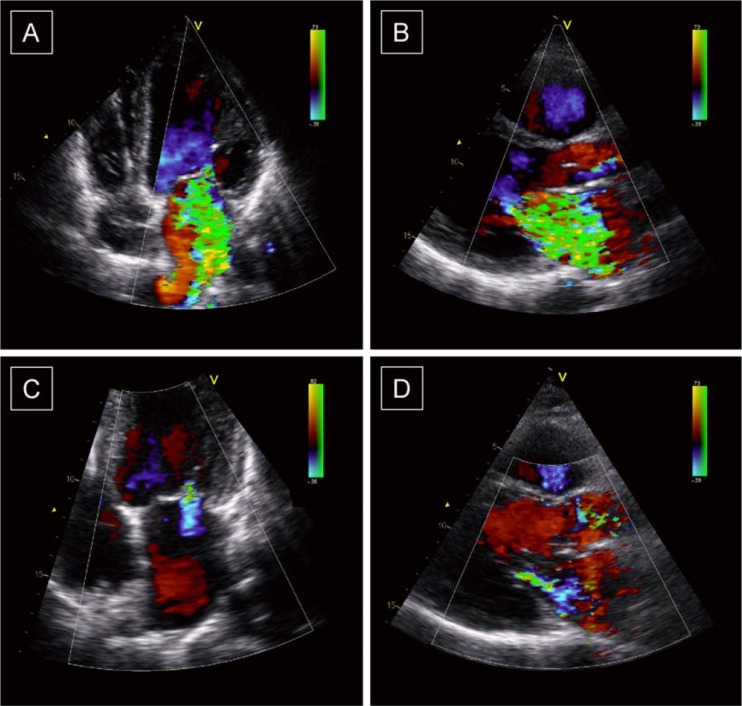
Example of a patient with a significant improvement in mitral regurgitation 6 months after cardiac resynchronization therapy. At baseline, the MR jet area exceeded 80 % of the left atrium area and the effective regurgitant orifice area (EROA) was 0.61 cm^2^ (Panels A [4-chamber view] and B [parasternal long-axis view]). At 6-month follow-up, the jet area decreased to 10 % of the left atrium area and the EROA was 0.19 cm^2^ (Panels C [4-chamber view] and D [parasternal long-axis view])
